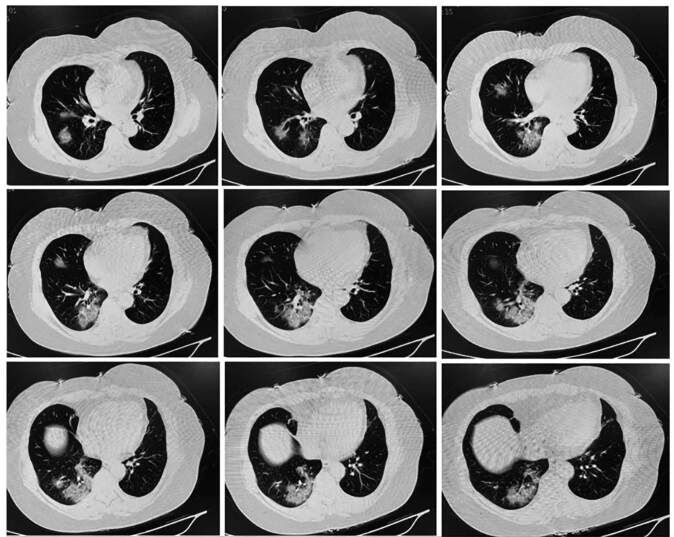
Chest CT image of the patient at admission. Chest CT showed patchy exudates in the lower lobe of right lung, slight fibrosis in the middle lobe of right lung and upper lobe of the left lung, nodules in the upper lobe of the right lung and lower lobe of the left lung and aortic sclerosis.
